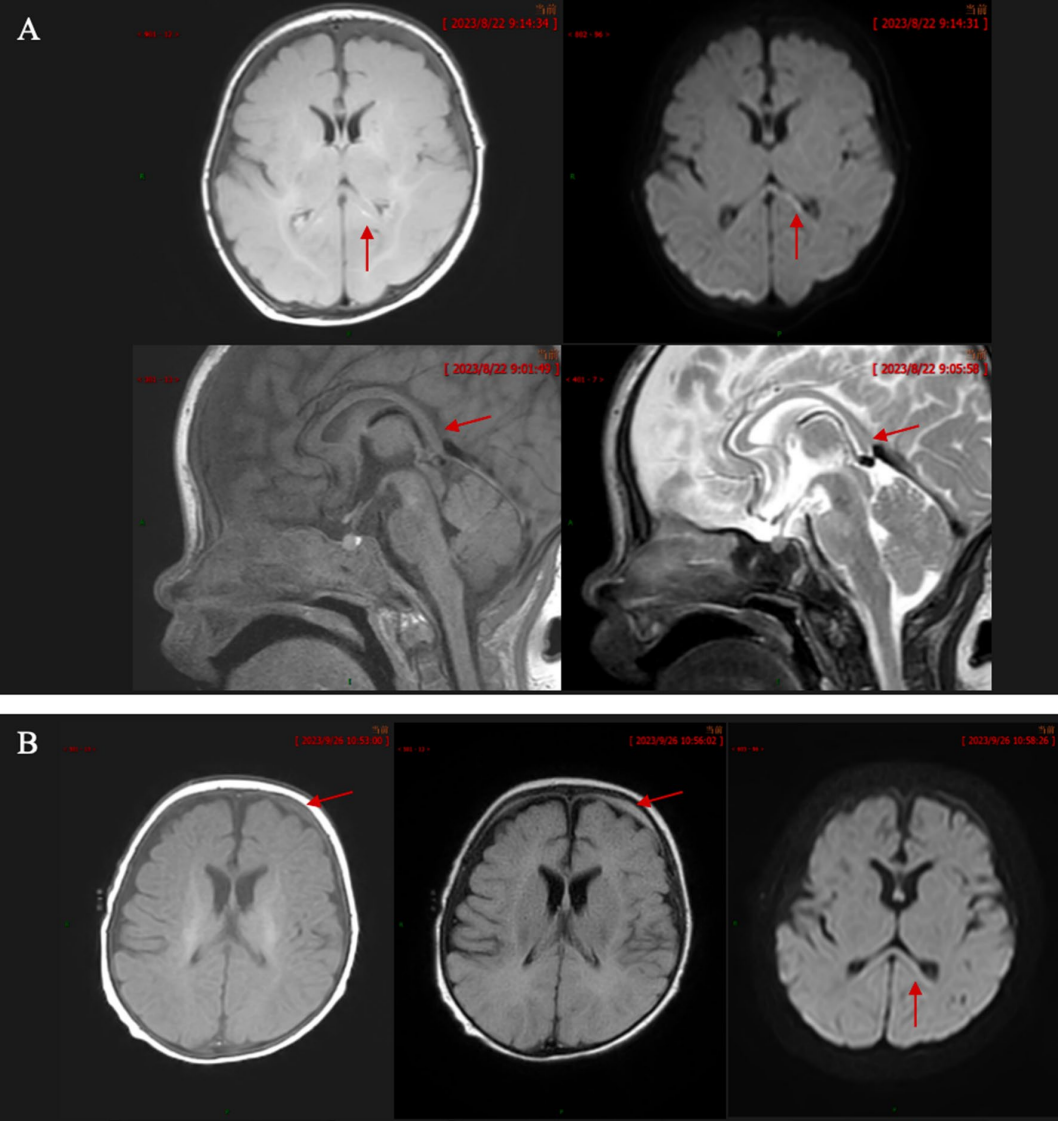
Cephalic MRI. A MRI examination on the 4th day after admission. The FLAIR sequence on the left side of the splenium of the corpus callosum showed a slight thickening, and a strip of high signal can be seen behind the splenium; DWI shows a high signal; T1 sagittal view shows a strip of low signal on the splenium of the corpus callosum. The T2 sagittal view shows high signal intensity in the splenium of corpus callosum; B MRI reexamination after 5 weeks of treatment. A strip of T1 signal is seen under the left frontal skull plate, the local signal in the FLAIR sequence is slightly higher, and the high signal in the splenium of the corpus callosum disappeared on DWI
